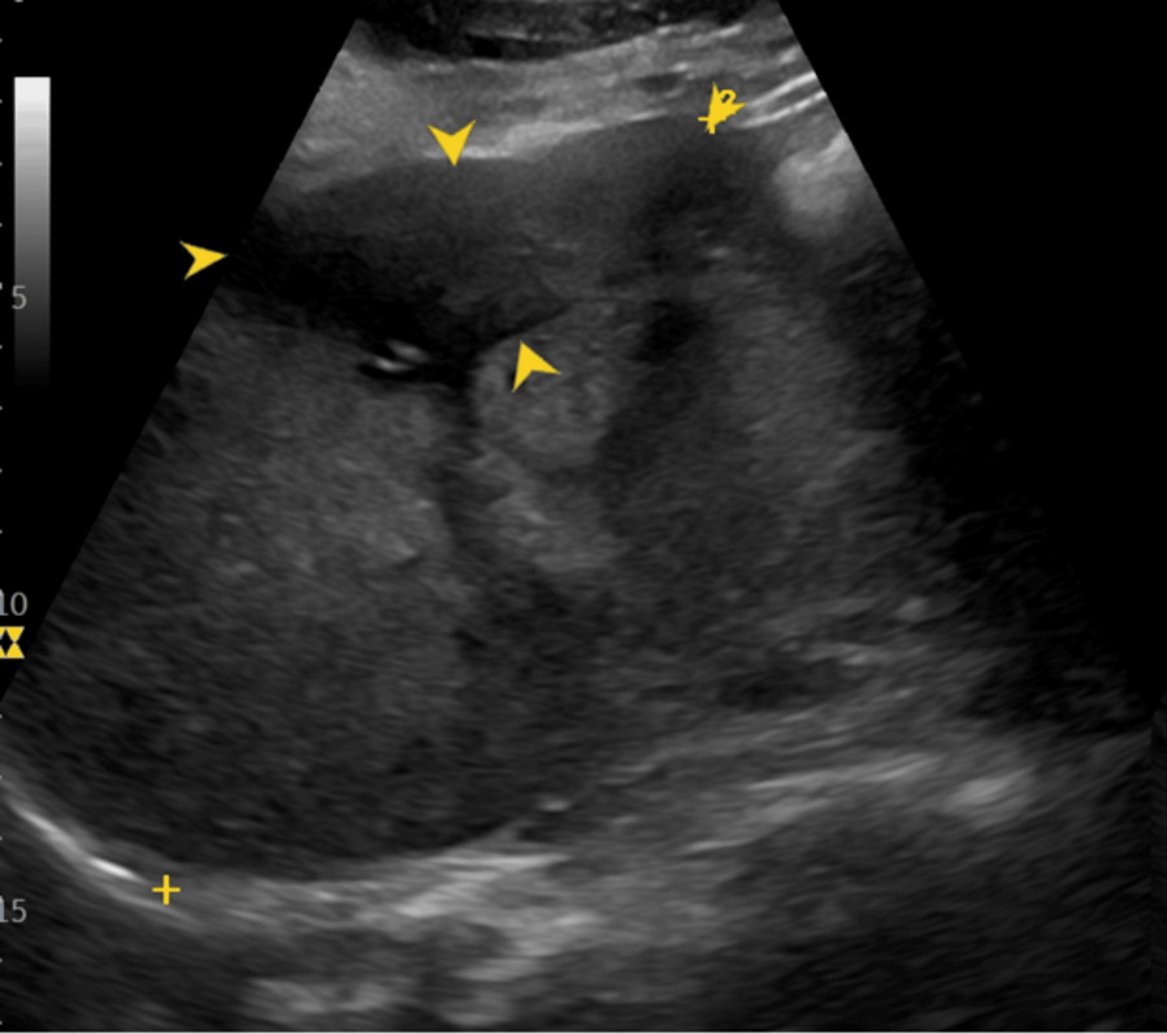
Gray-scale ultrasound image This demonstrates a large, hypoechoic liver lesion at a subscapular peripheral location annotated by the yellow arrows.
